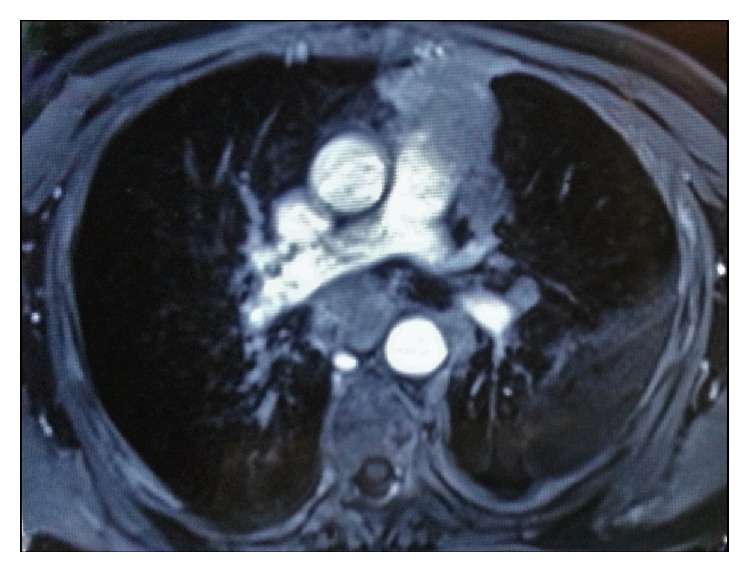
MRI chest revealing an anterior mediastinal mass which is infiltrating the surrounding lung parenchyma.
